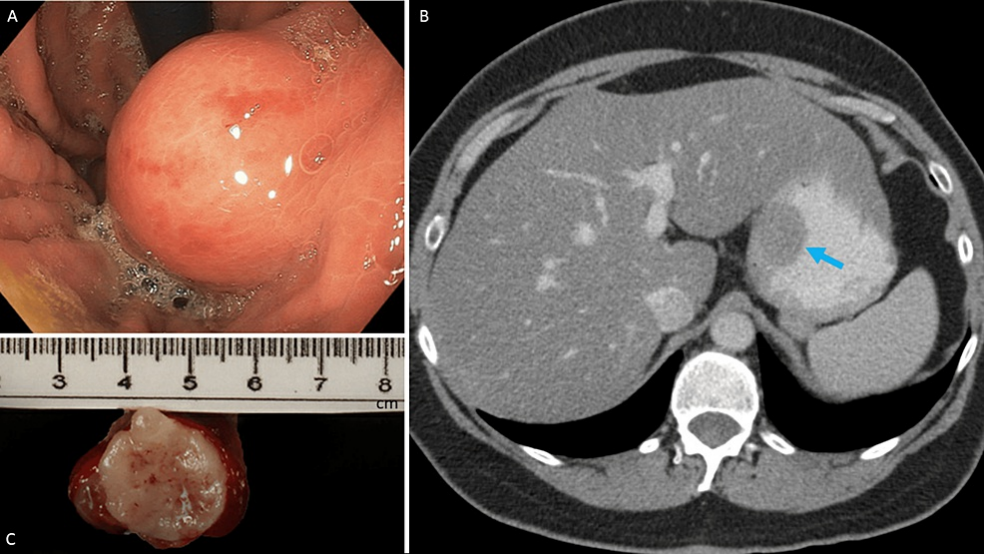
Mass in the gastric fundus/cardia region. (A) A submucosal lesion was revealed in EGD; (B) CT scan indicated an ovoid intraluminal mass. blue arrow: mass, approximately 3.0 cm × 2.3 cm; (C) the gross appearance of gastric leiomyosarcoma.
